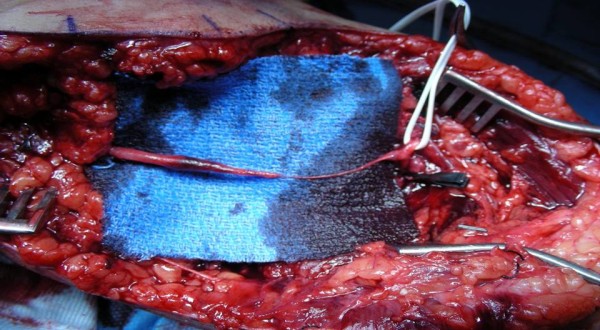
Same case as in figures 3, 4, 5. The intraoperative finding correlates to preoperative CT-angiography, revealing a brachial artery traction injury of about 5 cm length, located proximal to the bifurcation.
